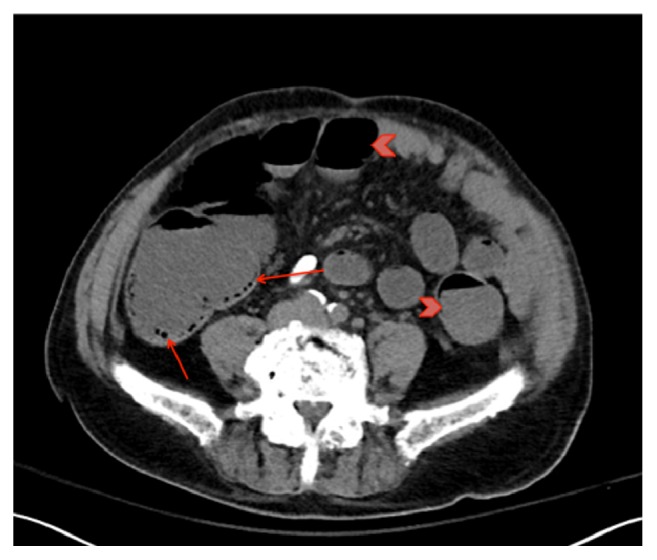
CT scan notable for colonic distention with submucosal and intraluminal gas (arrow), distended fluid-filled distal small bowel loops (arrowhead).
